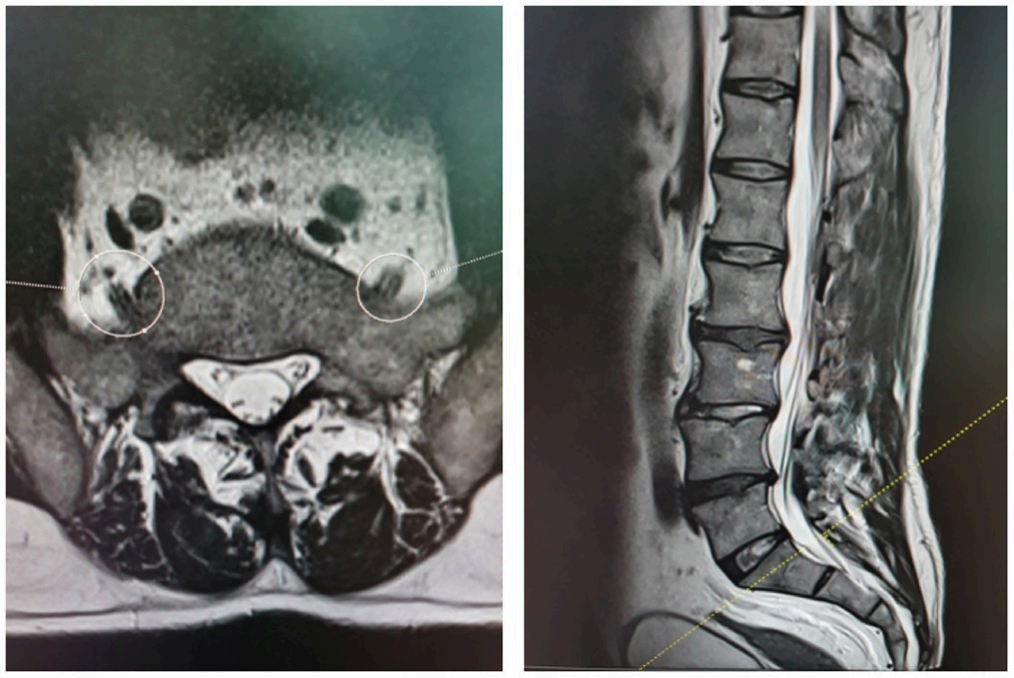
MRI showing transverse and sagittal projections with level of point A of SIJ.
